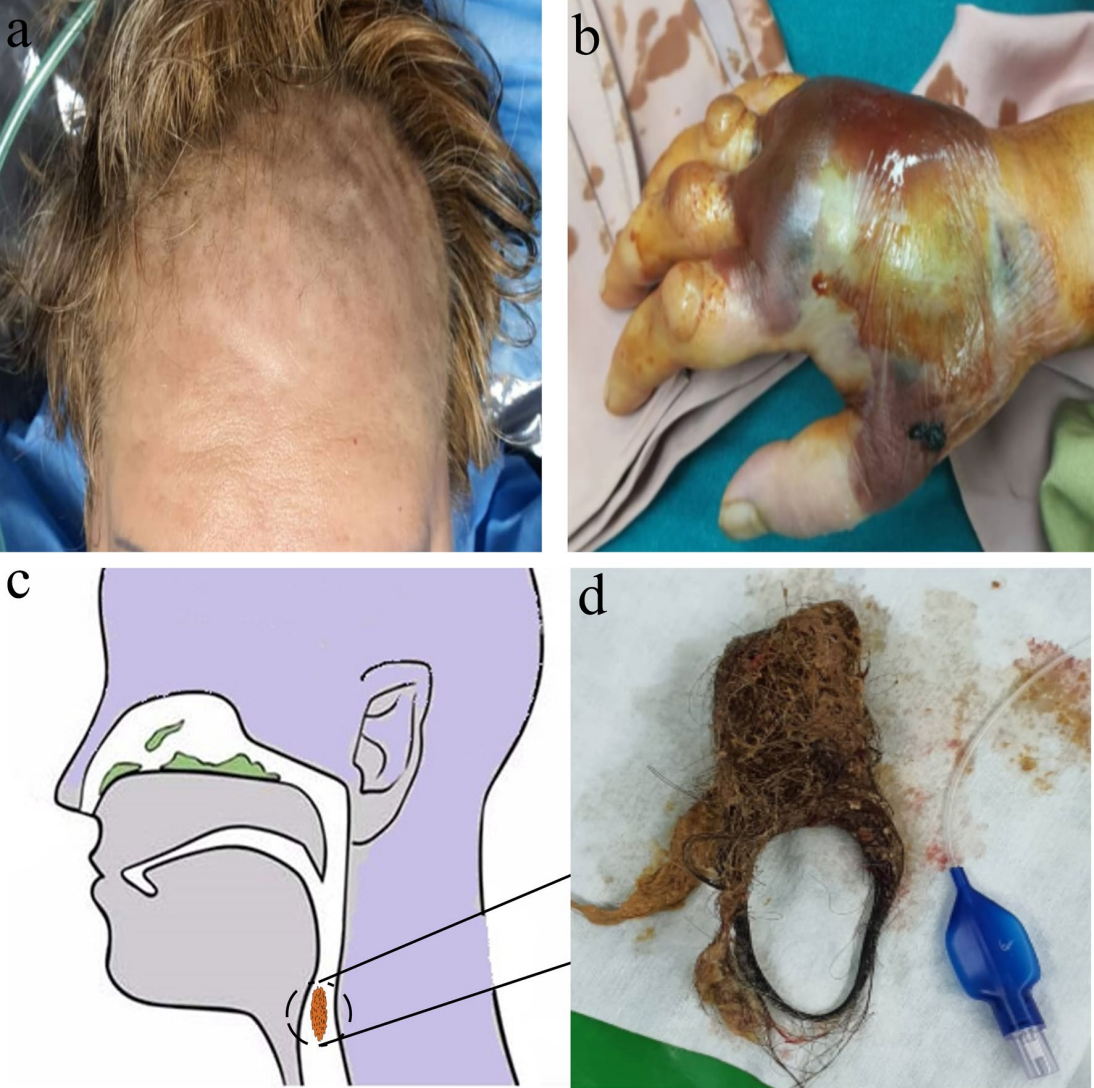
Rare case of 57-year-old woman with presentation of trichotillomania who suffered a leakage of the contrast agent from the vein under the skin, causing swelling of the hand during CT angiography of the lungs (a, b). Due to the lack of ventilation, laryngoscopy was performed and a foreign body in the subglottic area was detected (c). After removing it, trichobezoar be visualized on hypopharynx (d)
